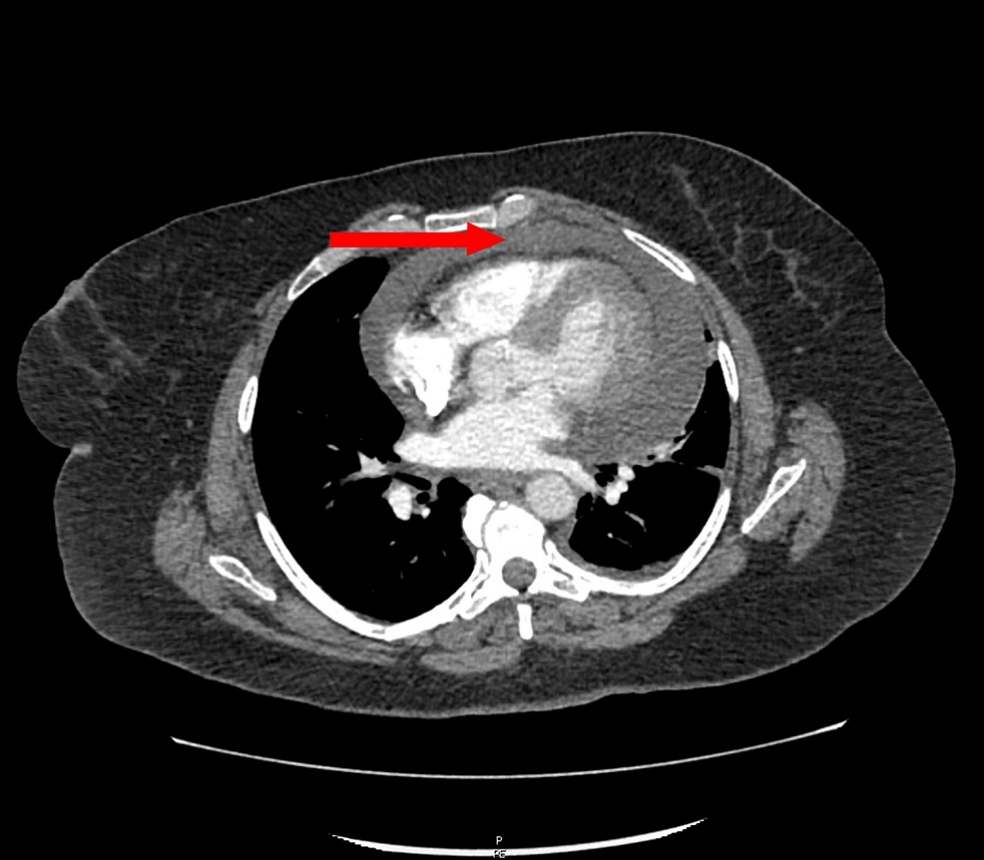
CT of the chest showing pericardial effusion (red arrow) CT: computed tomography
